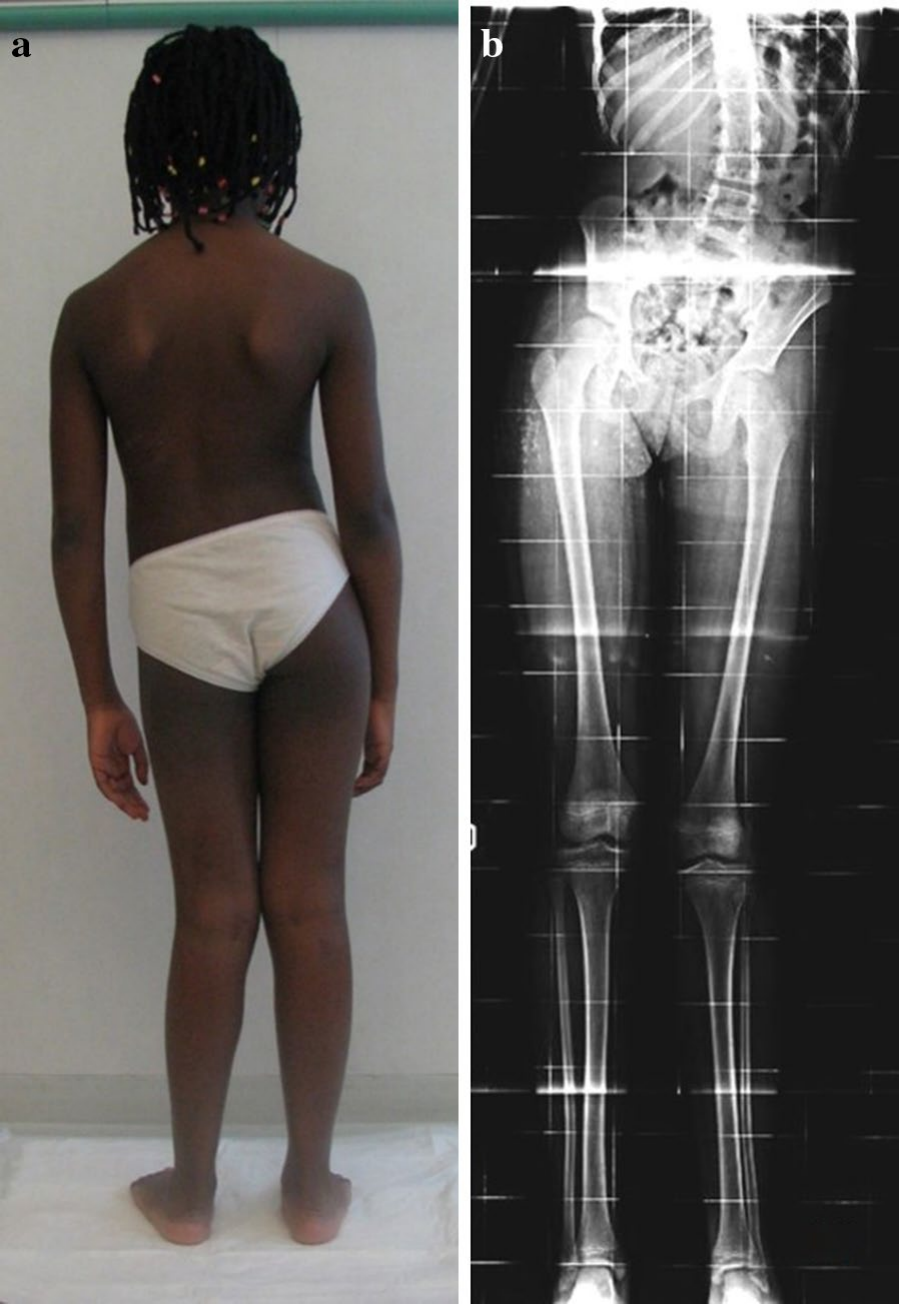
a, b Clinical and radiographic aspect of congenital lower limb length discrepancy in a 10-year-old patient. The length inequality was 5.2 cm for the left femur and 1.4 cm for the tibia
